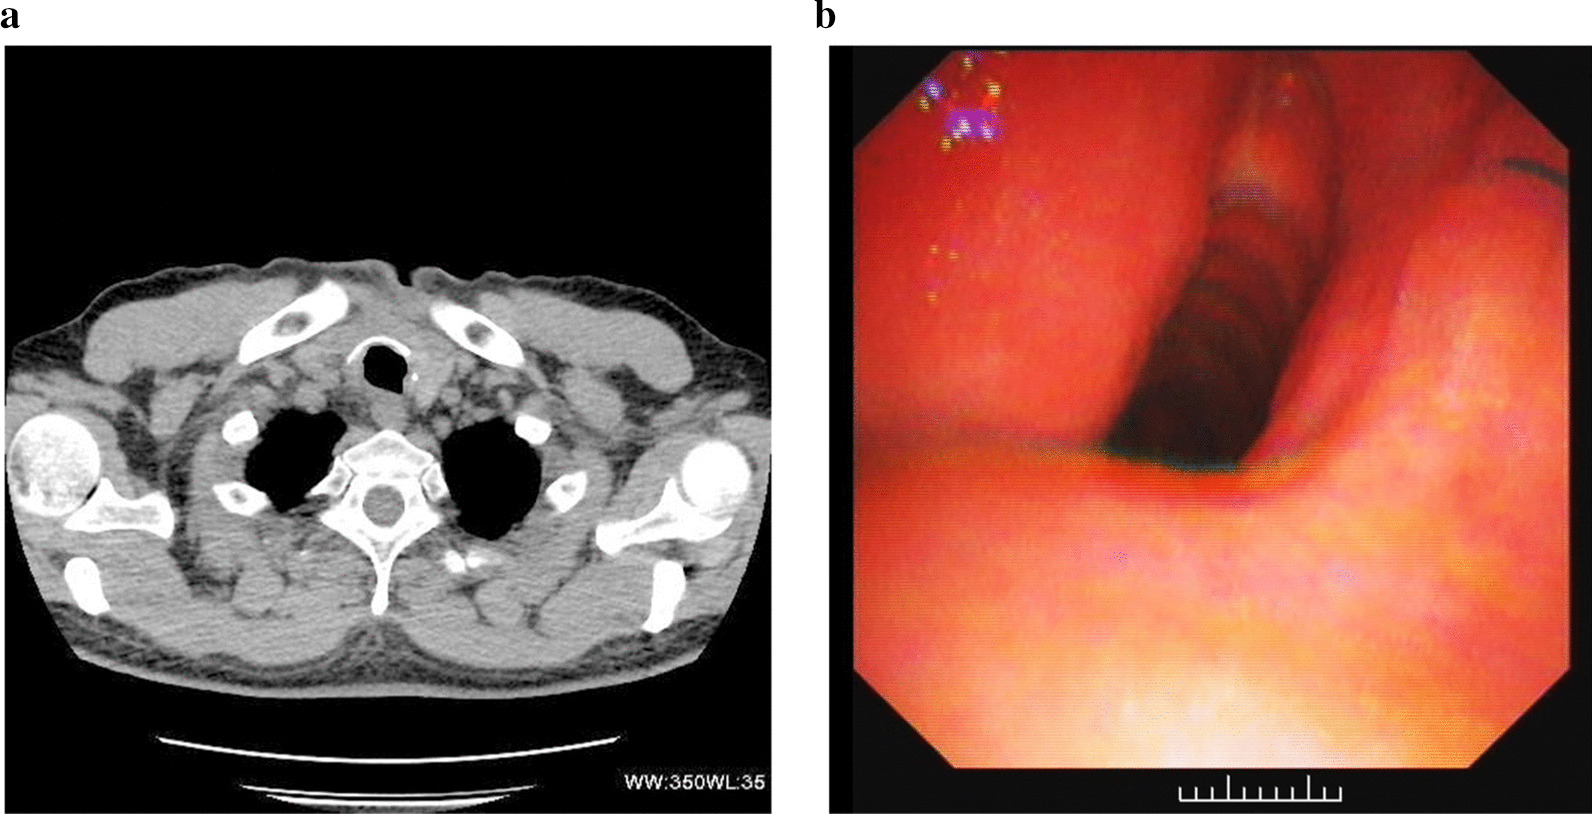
The patient again underwent CT examination and fiberoptic bronchoscopy one month after the operation. a An axial CT image shows that the tracheal cavity was regular after the original foreign body was removed. b Fiberoptic bronchoscopy reveals that the tracheal cavity was slightly narrow, but the tracheal mucosa was normal
